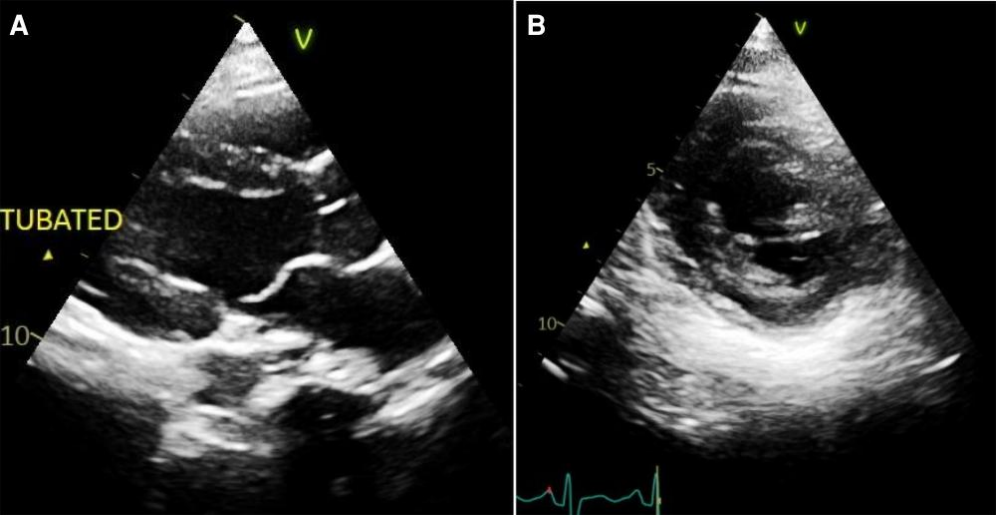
Transthoracic echocardiography demonstrating normal cardiac anatomy and function without evidence of structural heart disease: (*A*) parasternal long-axis view and (*B*) parasternal short-axis view.
